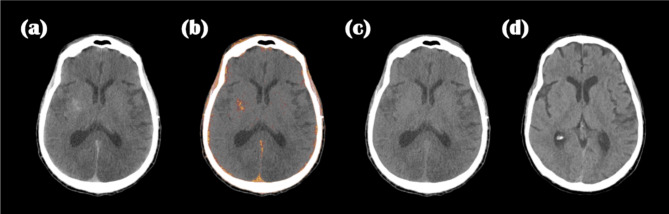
The neuro-images of patients with acute ischemic stroke treated using mechanical thrombectomy. (a) The original images of mobile PCD-CT, (b) iodine overlay map, (c) VNC image, and (d) follow-up non-contrast CT (NCCT) image. The threshold of VNC was set as 0.6.
